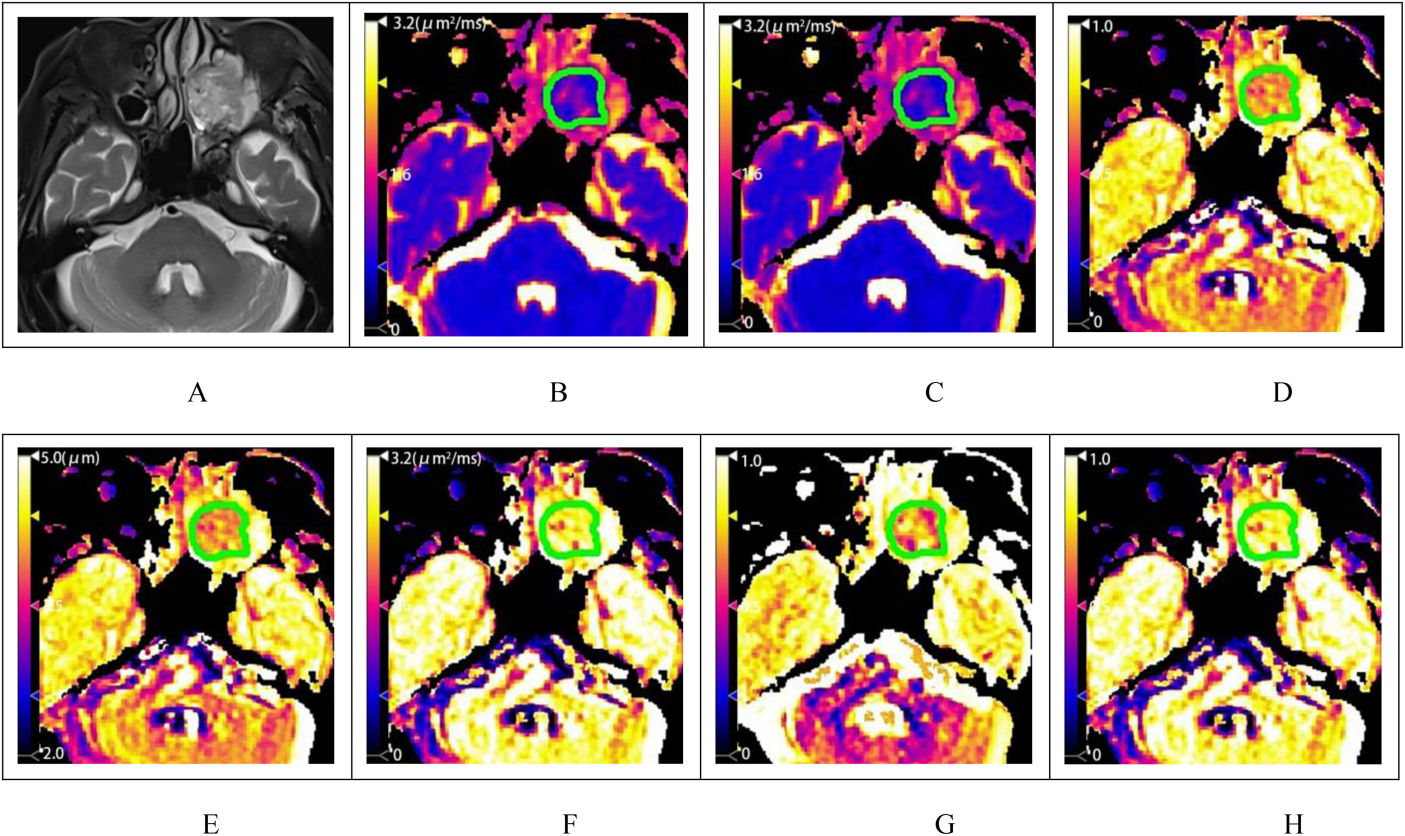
A patient with squamous cell carcinoma of the left maxillary sinus. (A) shows the T2-weighted fat-saturation image, and (B–H) display the pseudo-color maps of DWI, FROC, and CTRW model parameters, respectively. The measured values are as follows: ADC=0.963μm^2^/ms (B), D_FROC_=0.903μm^2^/ms (C), β_FROC_=0.780 (D), μ_FROC_=3.505μm (E), D_CTRW_=1.219μm^2^/ms (F), α_CTRW_=0.795 (G) and β_CTRW_=0.875 (3H).
